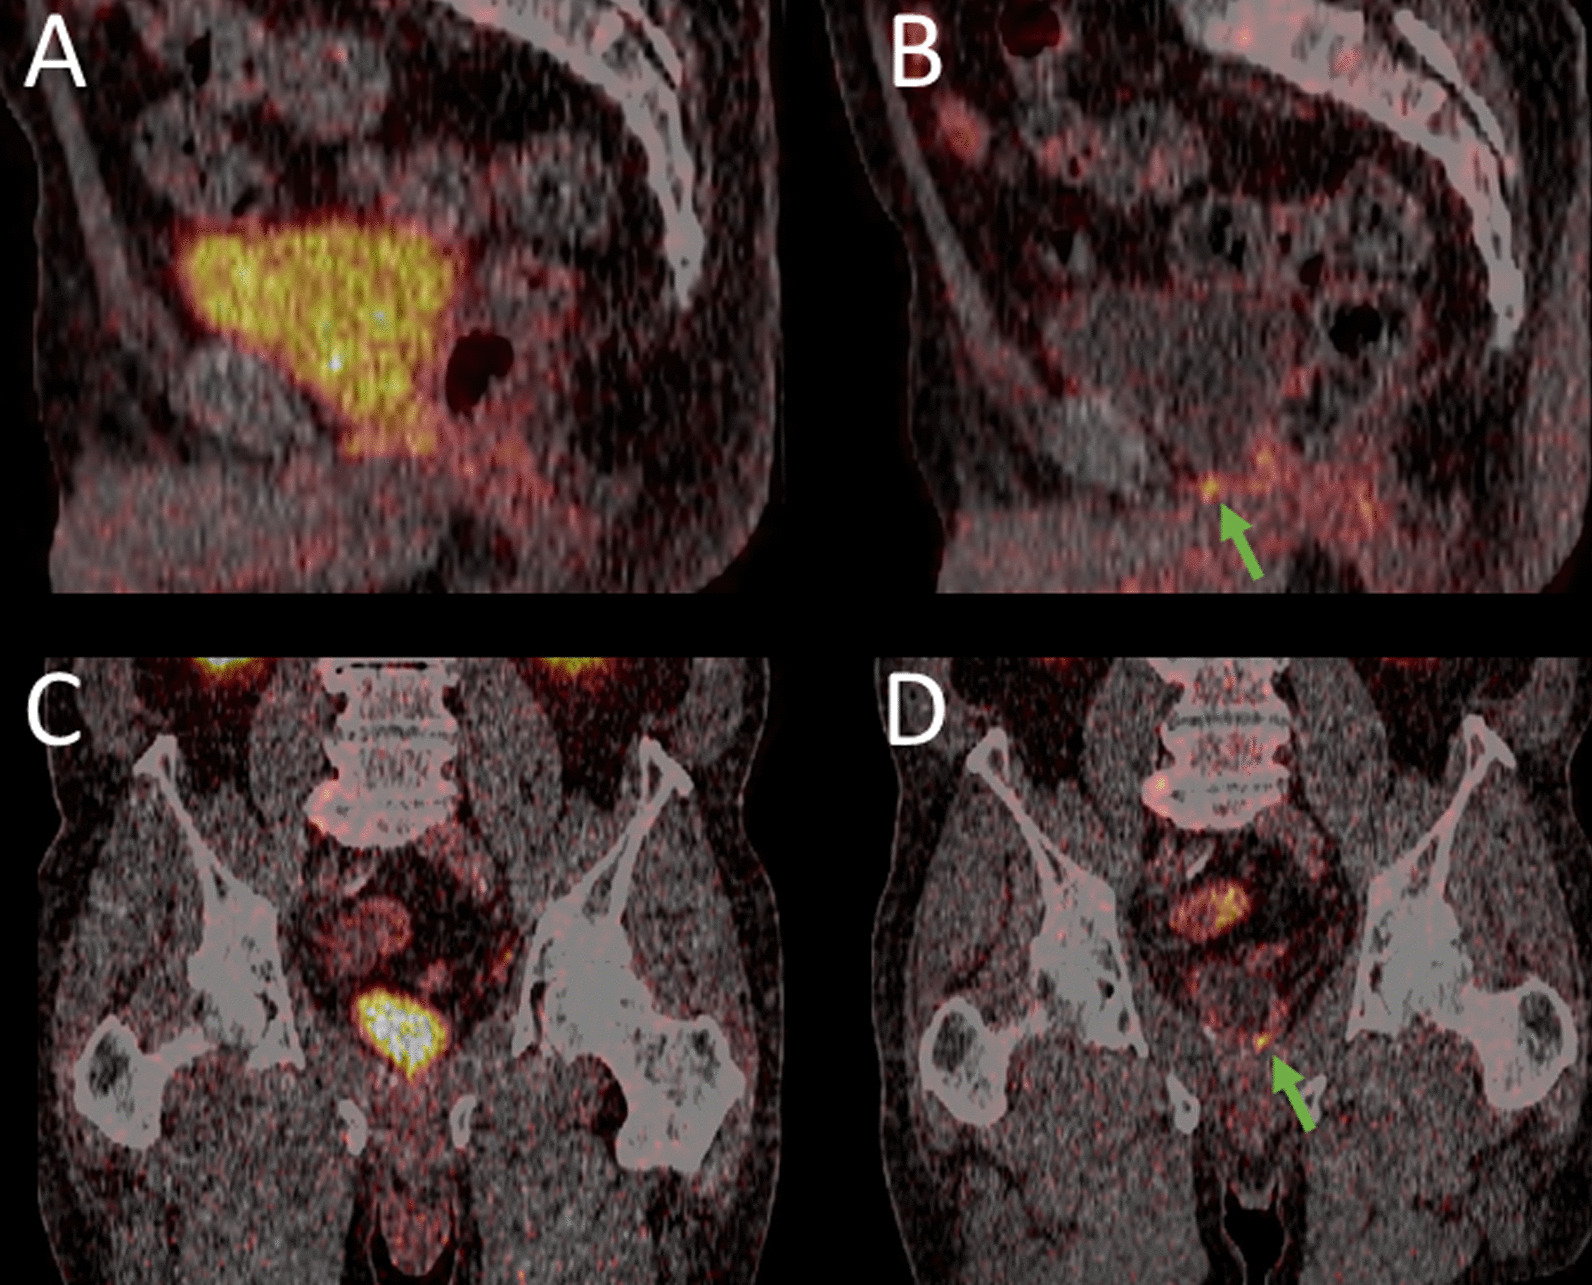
Sagittal fused [^68^Ga]-Ga-PSMA-11 (A) and [^18^F]-F-PSMA-1007 PET/CT (B) and coronal fused [^68^Ga]-Ga-PSMA-11 (C) and [^18^F]-F-PSMA-1007 PET/CT (D). While in [^68^Ga]-Ga-PSMA-11, there is only homogenous uptake in the prostatic fossa (A) clear focal uptake indicates local tumor recurrence in [^18^F]-F-PSMA-1007 PET/CT (green arrow)
